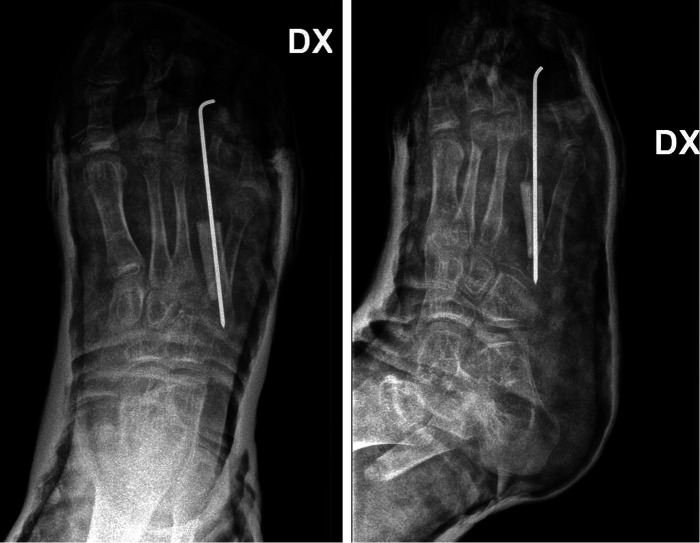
Post-operative x-ray A/P (A) and lateral (B) view showing reconstruction of IV metatarsal by fibular graft fixed to the base of V metatarsal (inverted Y shaped construct) using Kirschner’s wire and preserving tarso-metatarsal joint.
